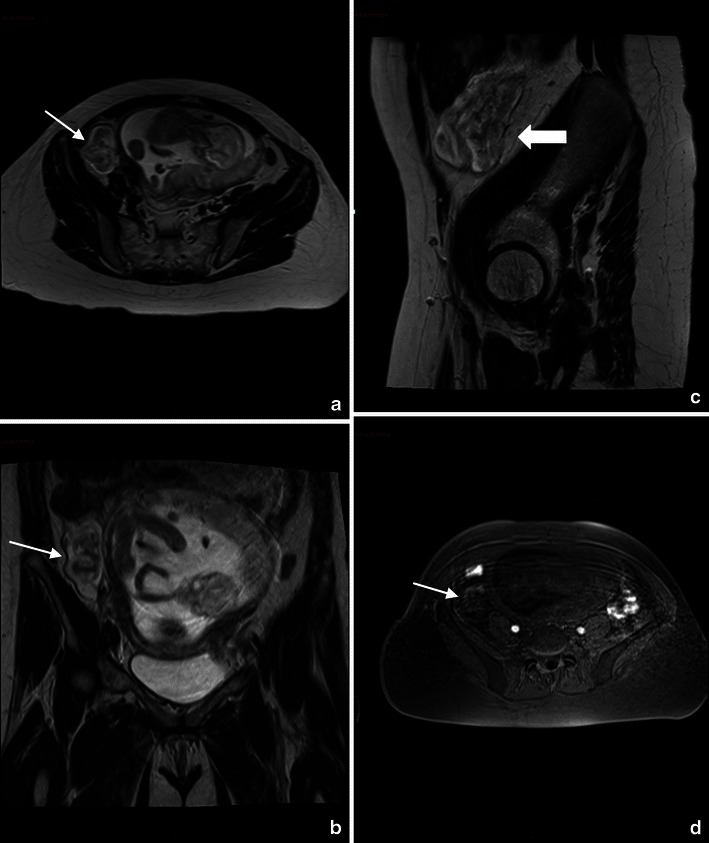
Ovarian torsion in a 38-year-old woman at gestation week 26 with acute pelvic pain. Axial (a), coronal (b) and sagittal (c) T2-weighted sequences show an enlarged, oedematous right ovary (arrow). Axial T1-weighted VIBE fat-saturated sequence (d) shows areas of hypersignal in the context of the right ovary indicative of haemorrhagic infarction
